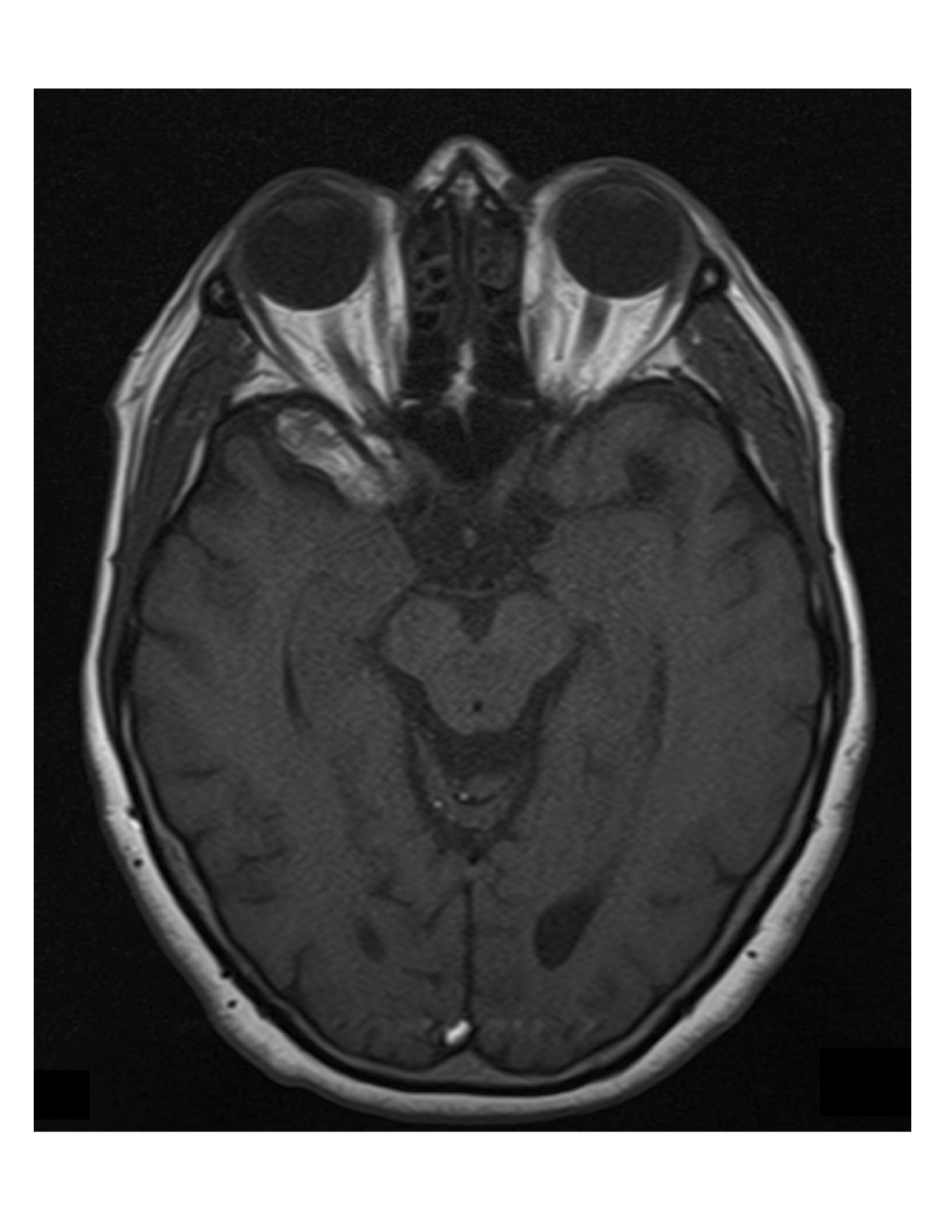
2014 Repeat MRI Repeat T1-weighted MRI image in 2014 showing no new evidence of significant change in appearance.
